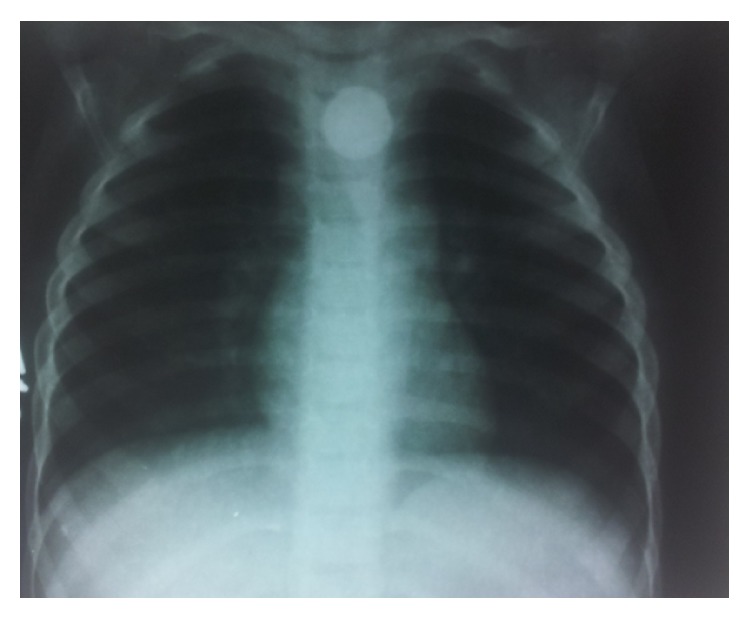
X-ray chest PA view showing a radio-opaque foreign body at the level of cricopharynx.
